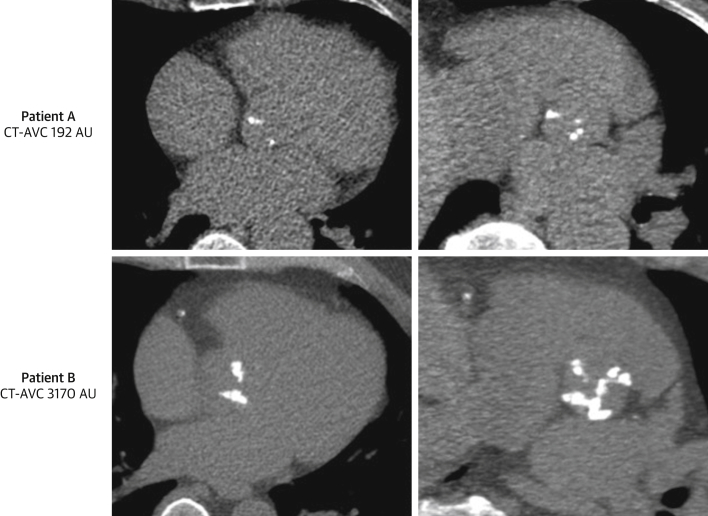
CT Calcium Scoring of the Aortic Valve An example of mild aortic valve calcification (AVC) (Patient A, top) and severe calcification by computed tomography (CT) (Patient B, bottom) in axial (left) and short-axis (right) views of the valve. AU = arbitrary unit(s).
